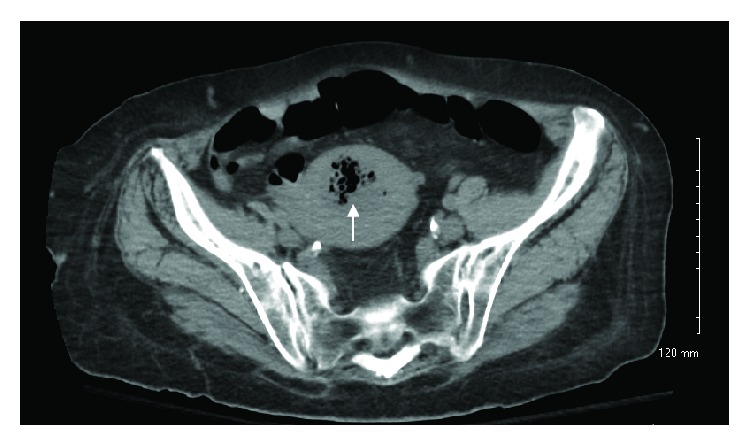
Axial CT image showing enlarged uterus with endometrial canal thickening and endometrial canal air (arrow) with surrounding inflammatory stranding, suggestive of endometritis.
